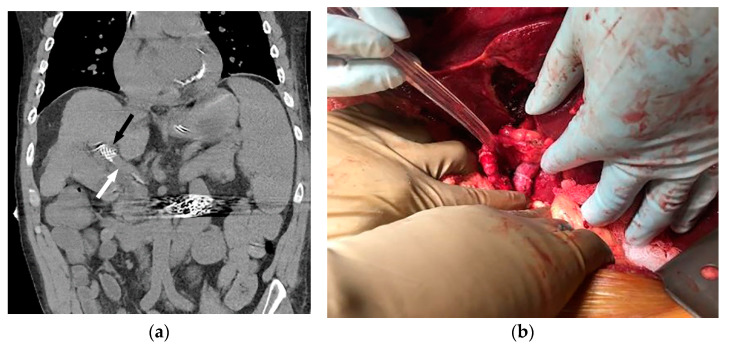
Coronal non-contrast CT (a) performed two days later (same patient as Figure 1 and Figure 2) demonstrates a larger-caliber portal vein (white arrow). The caudal aspect of the TIPS is noted (black arrow). The patient underwent successful orthotopic liver transplant approximately 1 year later (b), and remains well after 53 months of follow-up.
